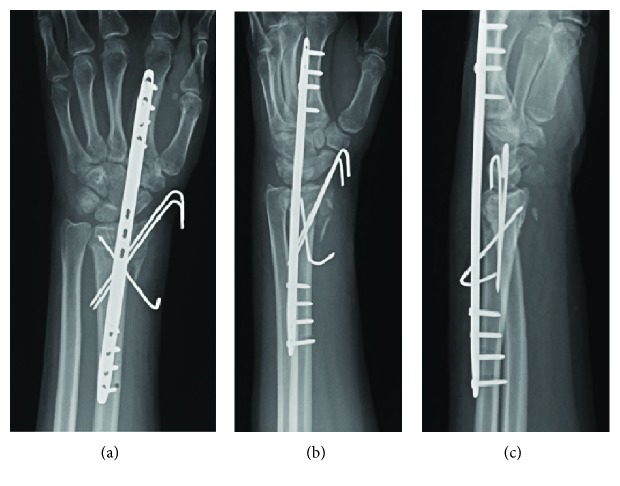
Initial fixation. Postoperative X-ray showing fixation with a 2.4/2.7 mm Synthes dorsal spanning plate and three K wires.
